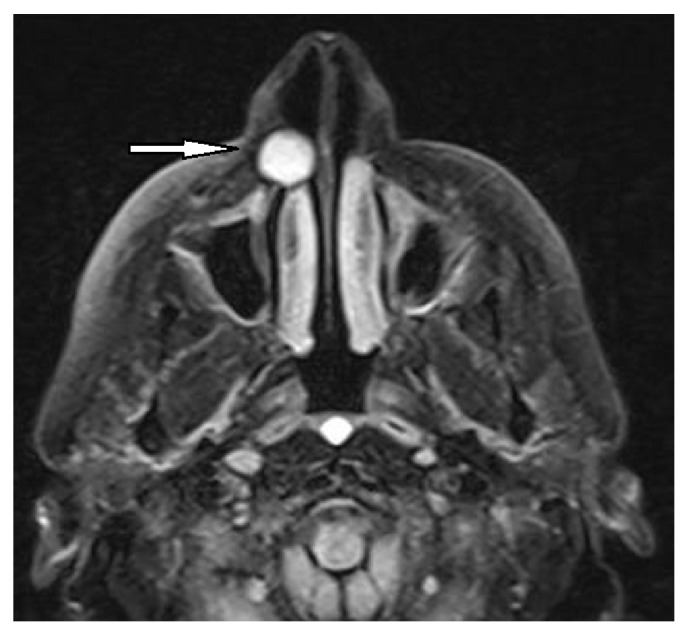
The axial T2-weighted fat-saturated image shows the well circumscribed lesion with a homogeneous hyperintensity (arrow).
